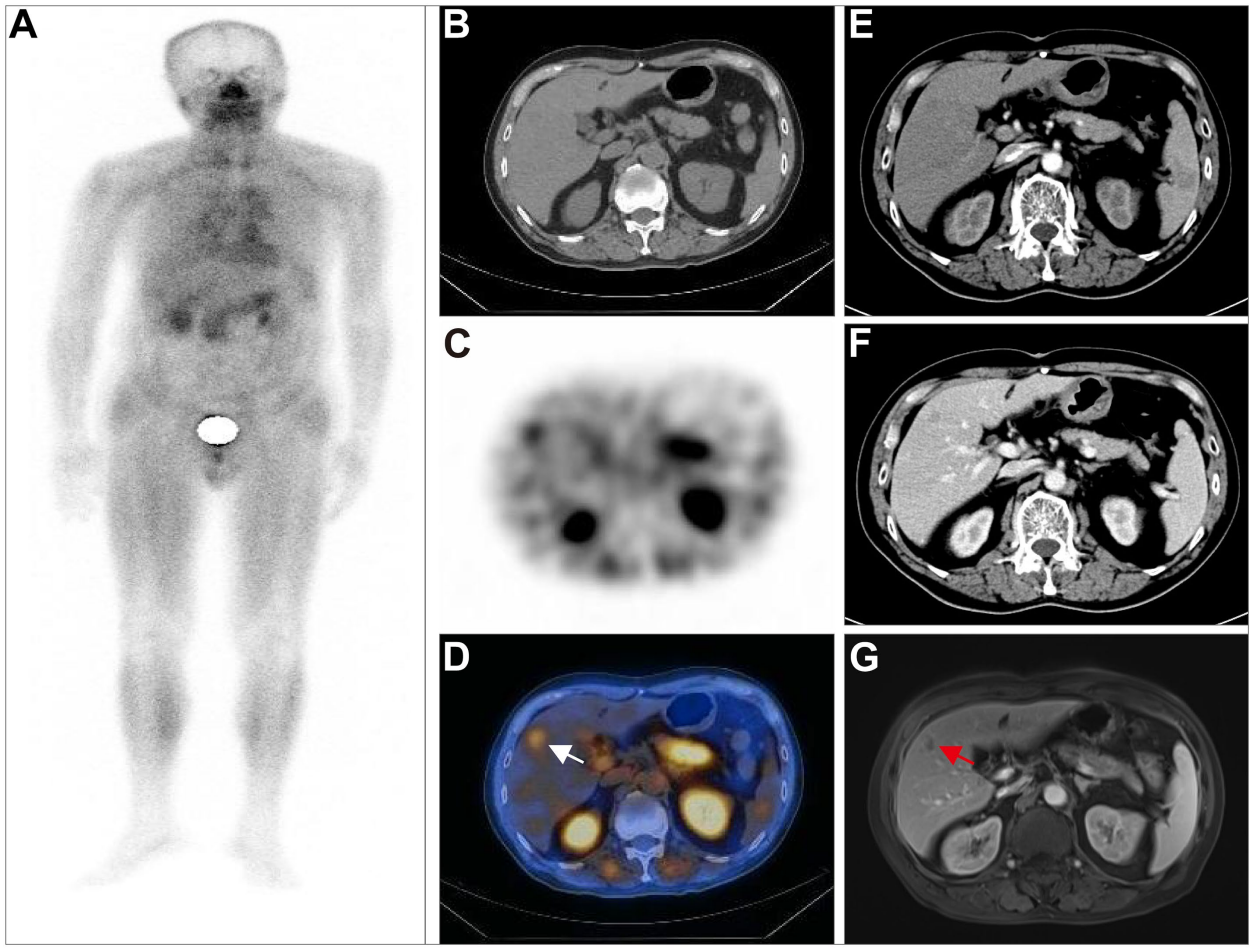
A 75-year-old postoperative male patient with transverse colon adenocarcinoma (pT3N0M0, stage IIA) underwent ^99m^Tc-FAPI-04 SPECT/CT, CECT examination, and MRI for follow-up evaluation. The whole-body MIP image (A). The axial CT image (B), axial SPECT image (C), and fused ^99m^Tc-FAPI-04 SPECT/CT image (D), where radiotracer uptake was observed in the anterior lobe of the liver, with a TBR of 4.26. CECT showed a small patchy low-density shadow in the right anterior lobe of the liver, with a mild enhancement at the edges in the venous phase and delayed phase (E,F). Although the lesion was suspicious, it could not be definitively diagnosed as liver metastases in CECT. The MRI examination (G) showed a nodule with low signal intensity on T1-weighted images and high signal intensity on T2-weighted images in the right anterior lobe of the liver. The lesion displayed peripheral ring-like but heterogeneous enhancement after administering the contrast agent, suggesting high chances of liver metastasis. The white arrow indicates high ^99m^Tc-FAPI-04 uptake in liver metastases, and the red arrow indicates the suspected metastatic lesion.
